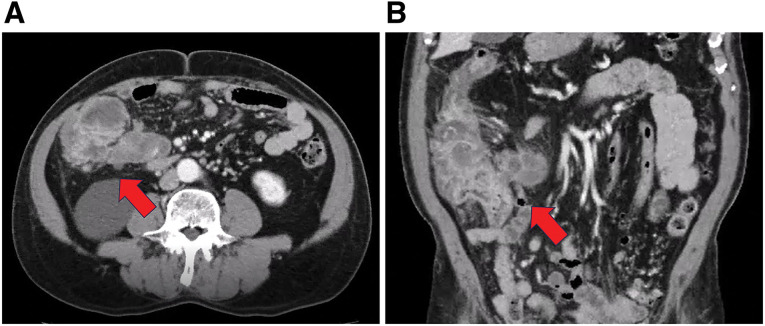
Preoperative contrast-enhanced CT findings. (A) Axial and (B) coronal contrast-enhanced CT images show a circumferential lesion in the ileocecal region (arrows) with enlarged regional lymph nodes, causing marked stenosis and raising suspicion for locally advanced colon cancer with acute obstructive symptoms.
